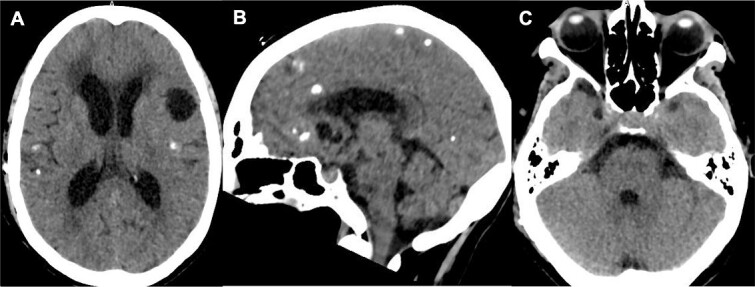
Initial brain CT revealed multiple calcified cysts with ventriculomegaly in the axial (A, C) and sagittal (B) views.
